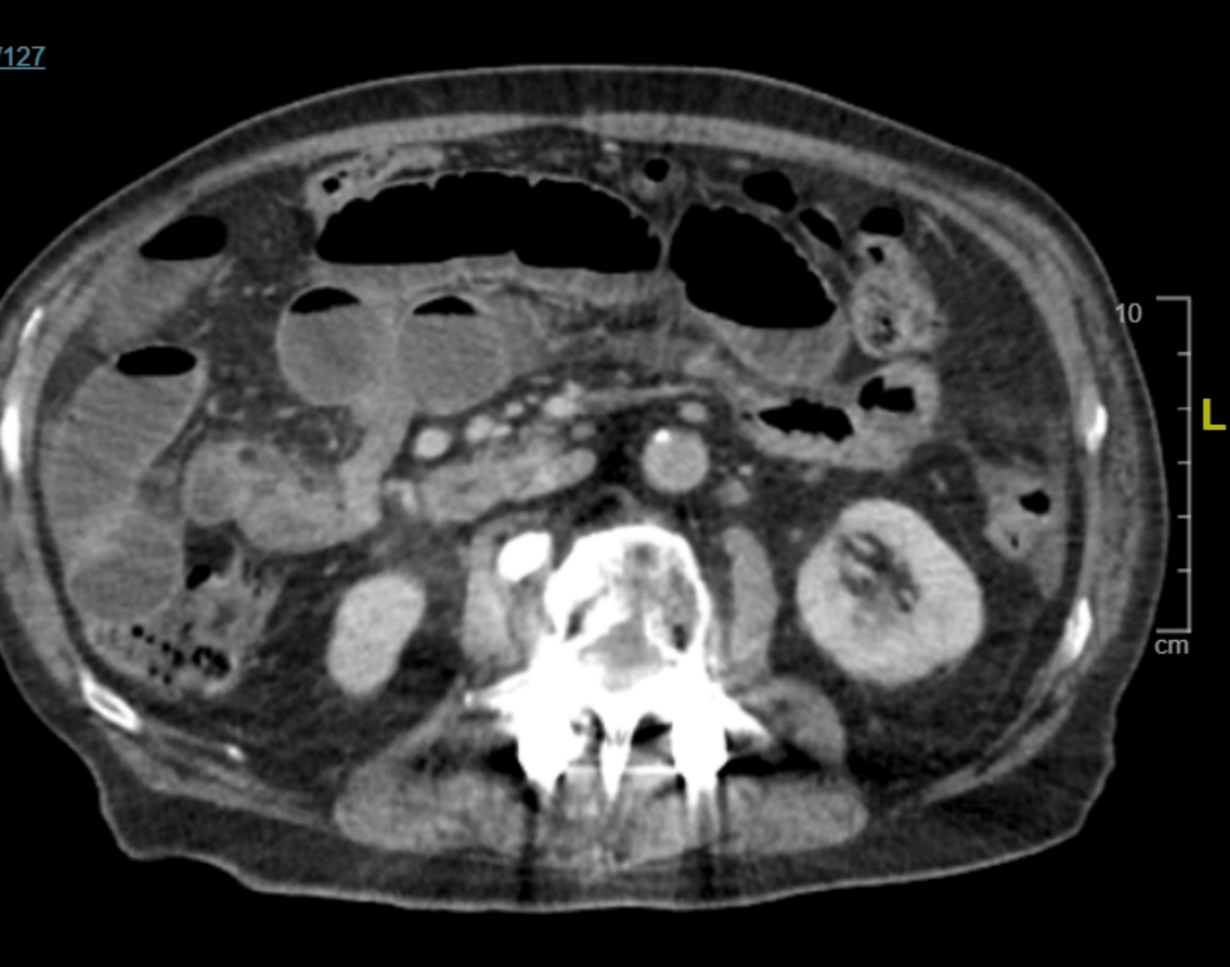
Representative CT image from a patient presenting with SBO SBO, small bowel obstruction
